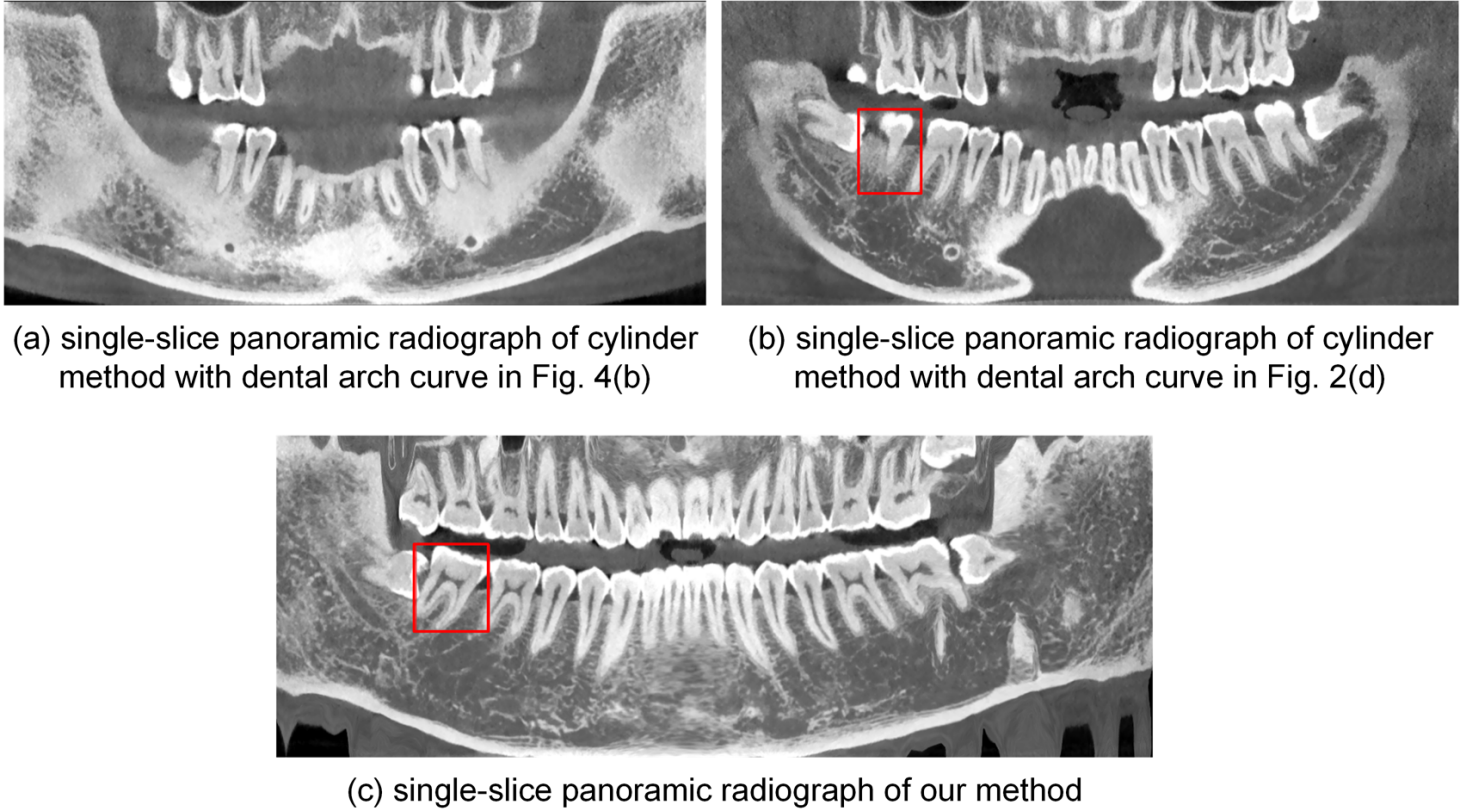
Single-slice panoramic radiographs by different methods from dataset 1.
